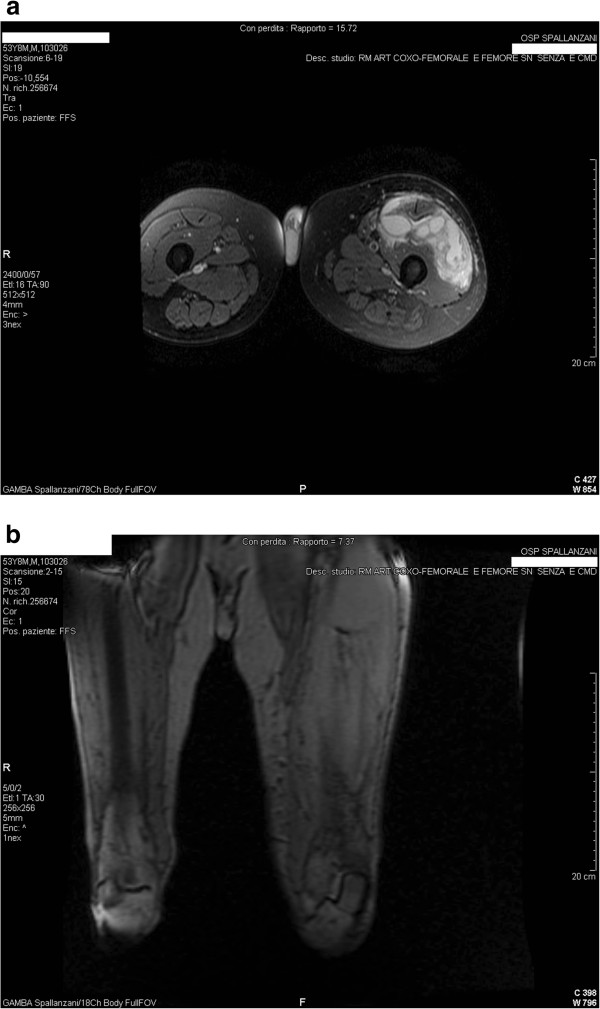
Magnetic Resonance Imaging of left thigh at admission. a. T1 after contrast coronal image showing hyperintense necrotic lesion of the left thight; b. T2 FLAIR weighted sagittal image of the same lesion that appear hypointense.
